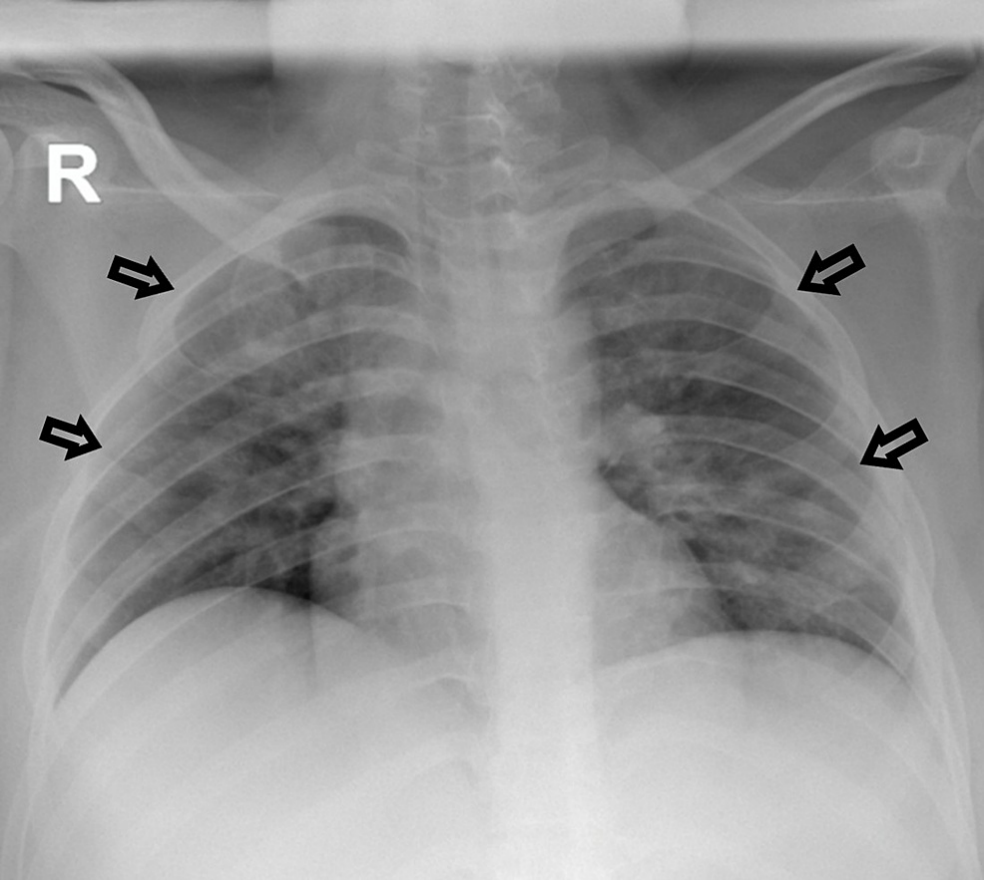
Chest x-ray of case 2 showing bilateral infiltrates consistent with COVID-19 pneumonia.
